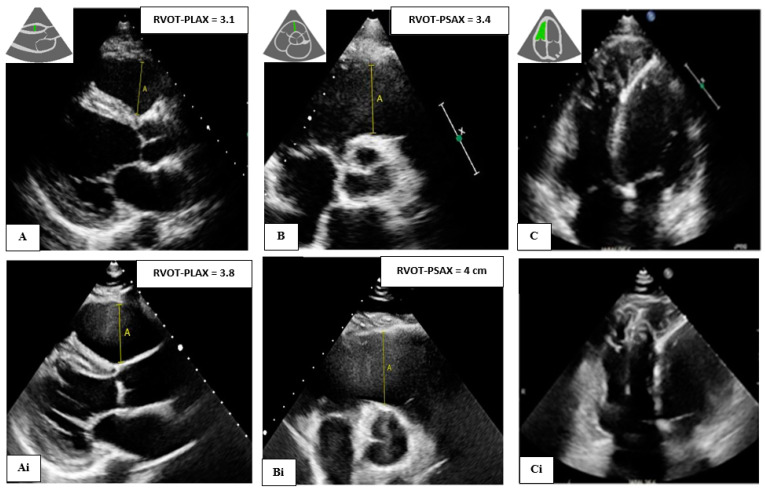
A 39-year-old male with *PKP2* pathogenic variant and definite ARVC (Top) Baseline images showing RVOT (still normal) in (A) parasternal longs axis (PLAX), (B) parasternal short axis (PSAX), and (C) apical four chamber view showing dilated and trabeculated right ventricle (RV). Further dilation was observed after 6 years of follow-up (Ai,Bi,Ci) (bottom).
